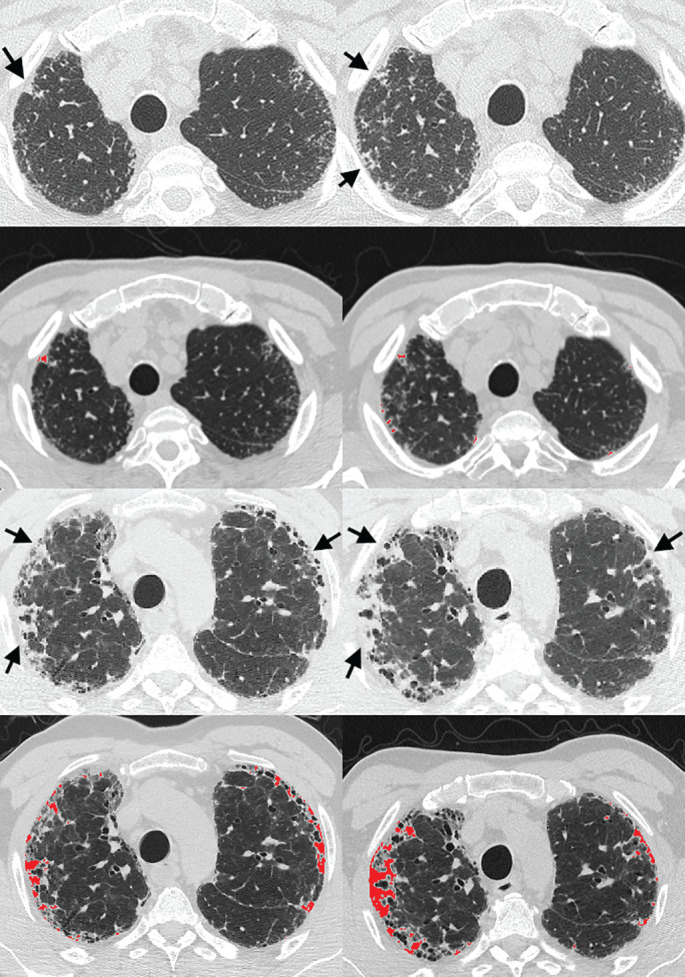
Visual characteristics of progressive pleuroparenchymal fibroelastosis (PPFE). Baseline (left column) and follow-up (right column) computed tomography (CT) scans in patients diagnosed with idiopathic pulmonary fibrosis by multidisciplinary team. The top two rows show axial CT images of the upper zones taken 13 months apart in a 73-year-old male with normal baseline forced vital capacity (FVC) (83%) and diffusion capacity of the lung for carbon monoxide (*D*_LCO_) (74%). Arrows (top row) show areas of PPFE increasing in extent on the second CT, also highlighted by image overlays of PPFE regions (second row). The bottom two rows show axial CT images taken 9 months apart in a 54-year-old male with abnormal baseline FVC (46%) and *D*_LCO_ (35%). Arrows (third row) show more extensive PPFE proliferating dramatically on the second CT, again highlighted on image overlays of PPFE regions (bottom row).
